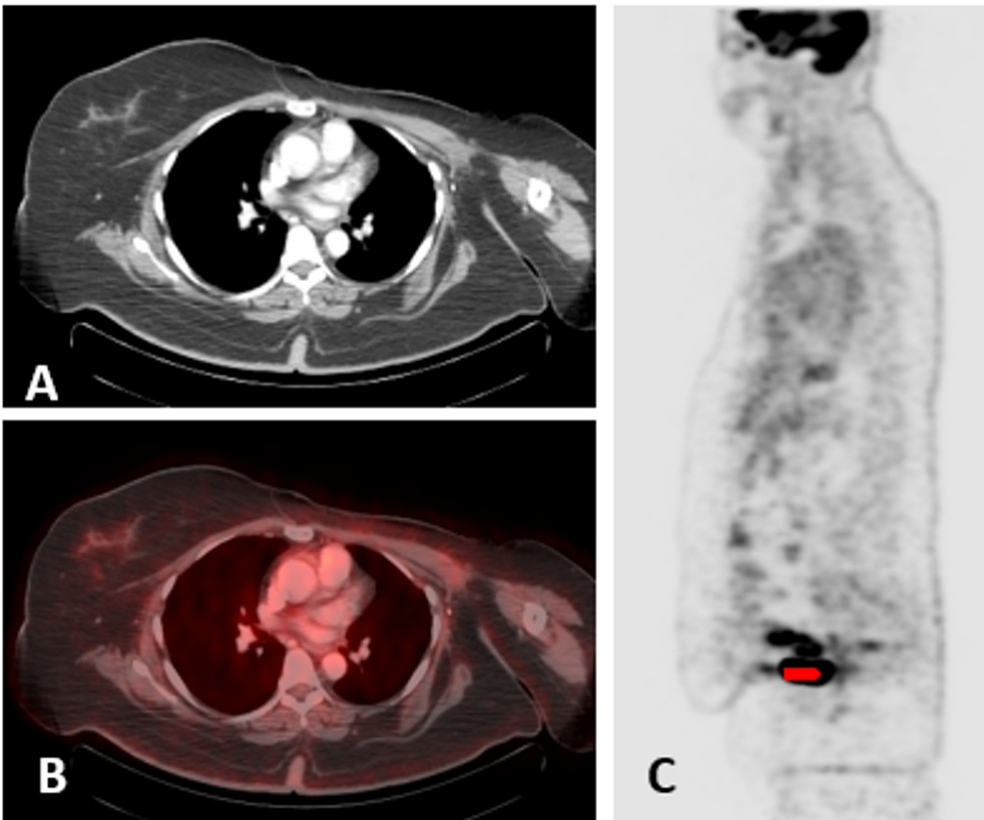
Axial CT/PET-CT images (A, B) show changes of left mastectomy without any local recurrence. Hypermetabolic endometrial lesions were noted on sagittal PET-only image (C) indicated by arrowhead.
